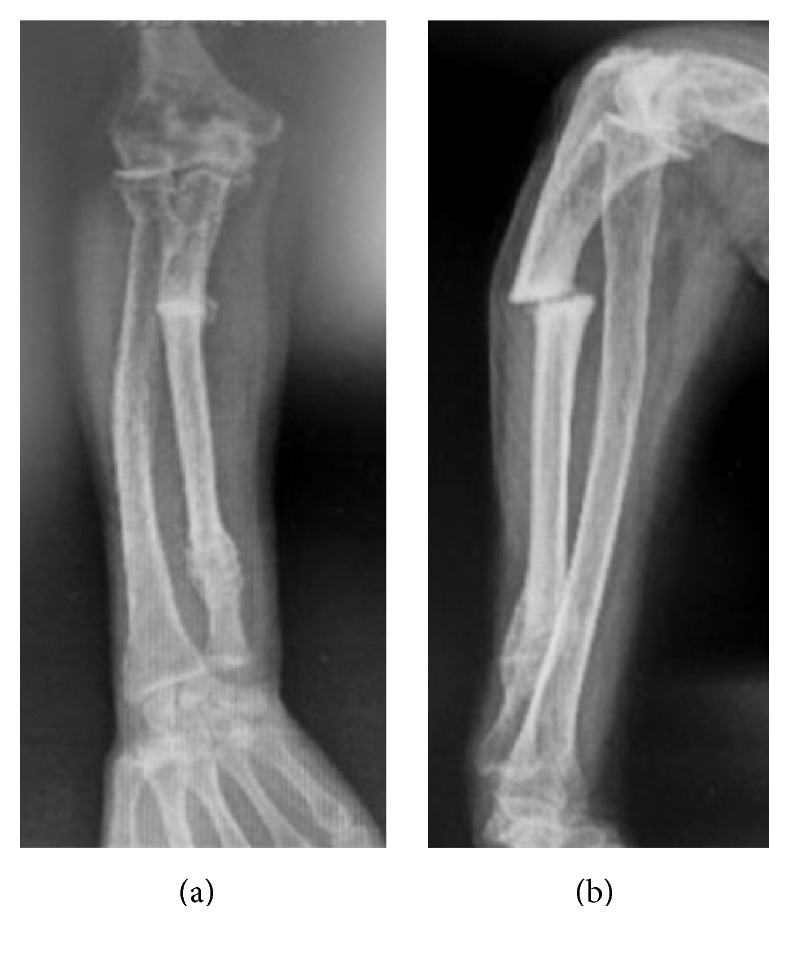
Forearm radiographs at the 6th week follow-up demonstrating the nonunion.
